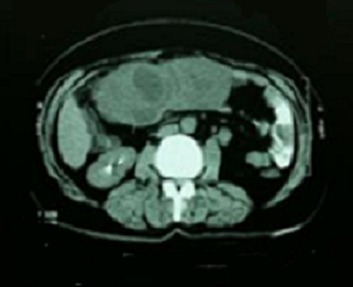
CT scan of the abdomen showing a round tissular mass with irregular edges with central necrotic areas enhancement in the peripheral zones
